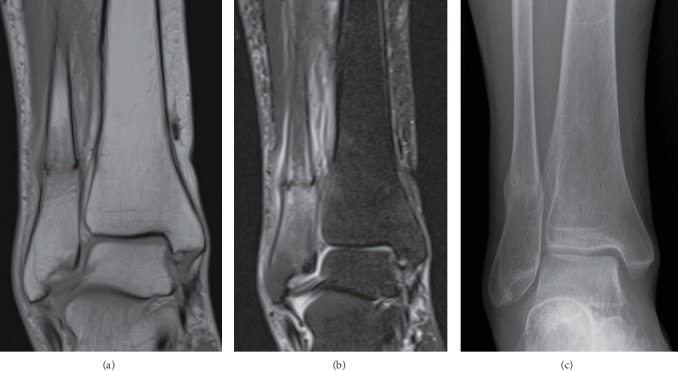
MRI showed an isolated fibular fracture, bone marrow edema, and surrounding soft tissue edema without any pathological lesion: (a) PDW coronal, (b) STIR coronal. Bone union was confirmed in anteroposterior radiograph (c) three months after identifying fracture.
